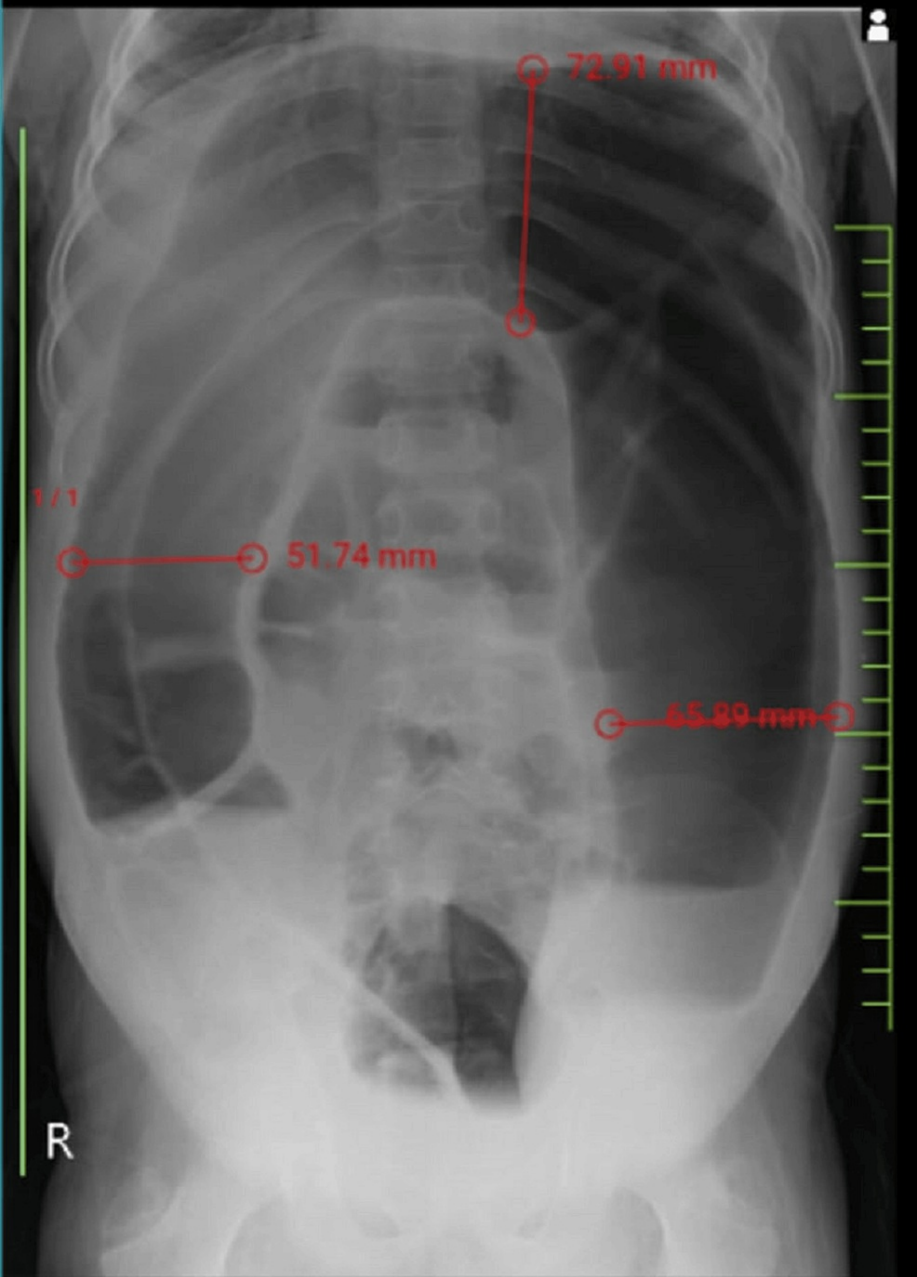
Abdominal radiograph showing dilated large bowel loops, with the largest dimension in the transverse colon noted as 72.91 mm
